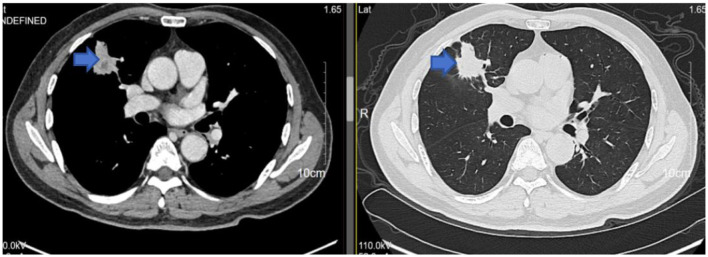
Chest CT scans on admission. The chest CT scan on admission (November 25) revealed spiculated mass in the right upper lobe with surrounding consolidation and minimal liquefactive necrosis.
